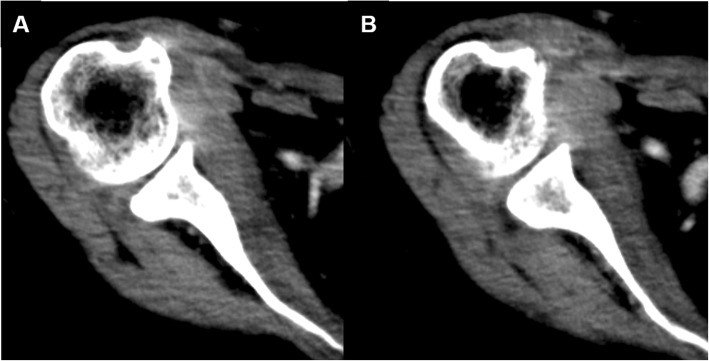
Contrast-enhanced CT imaging performed 2 months after surgery revealed no relapse of abscess (a and b)
